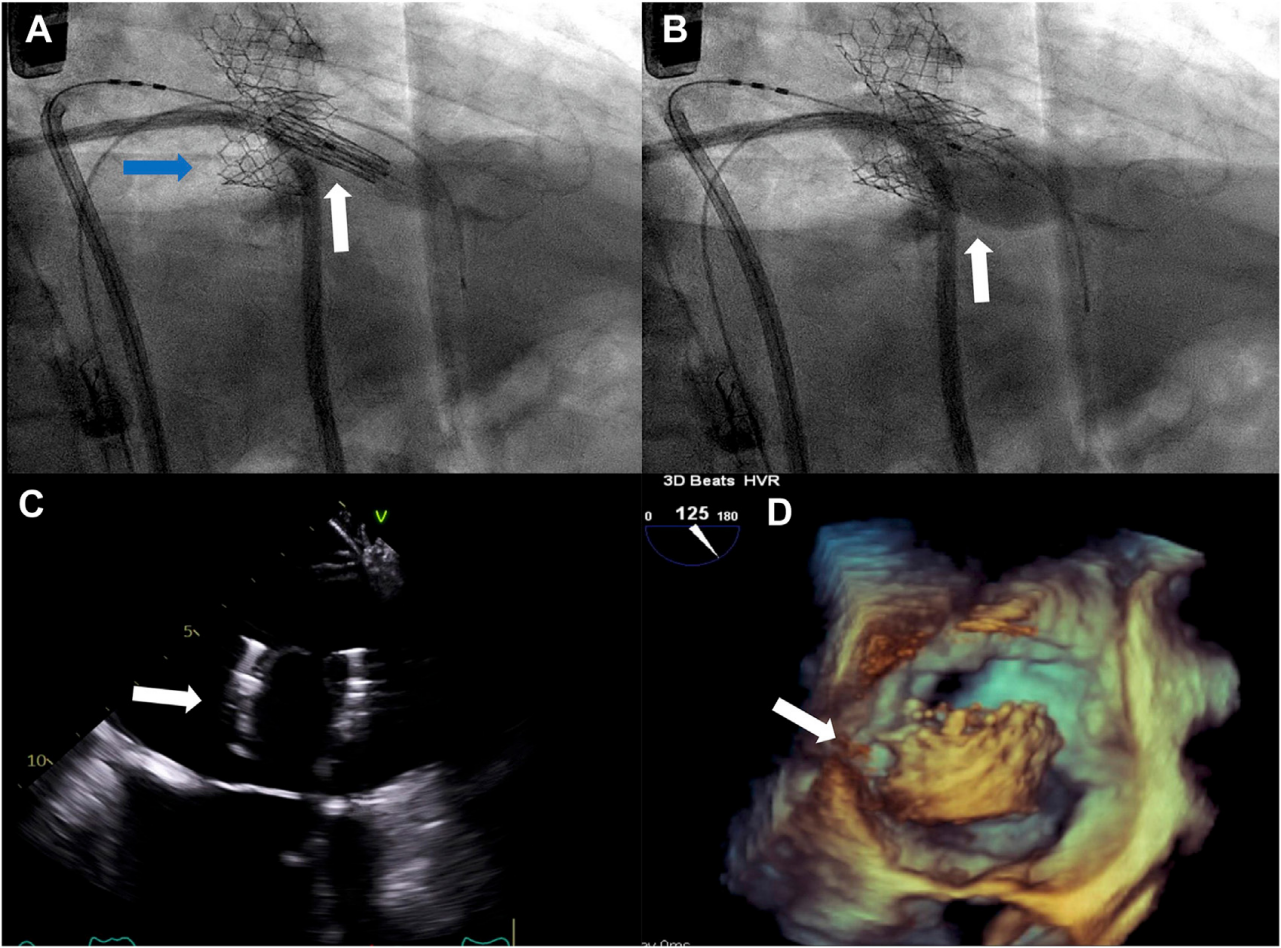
(A, B) Fluoroscopy in second valve delivery and deployment. (A) First valve in the mitral position but migration toward left atrium (blue arrow), second valve delivered in the valve-in-valve position (white arrow). (B) Deployment of second valve (arrow). (C, D) Intraprocedural transesophageal echocardiography after second valve deployment. Transcatheter mitral valve replacement valve embolized into left atrium (arrow).
